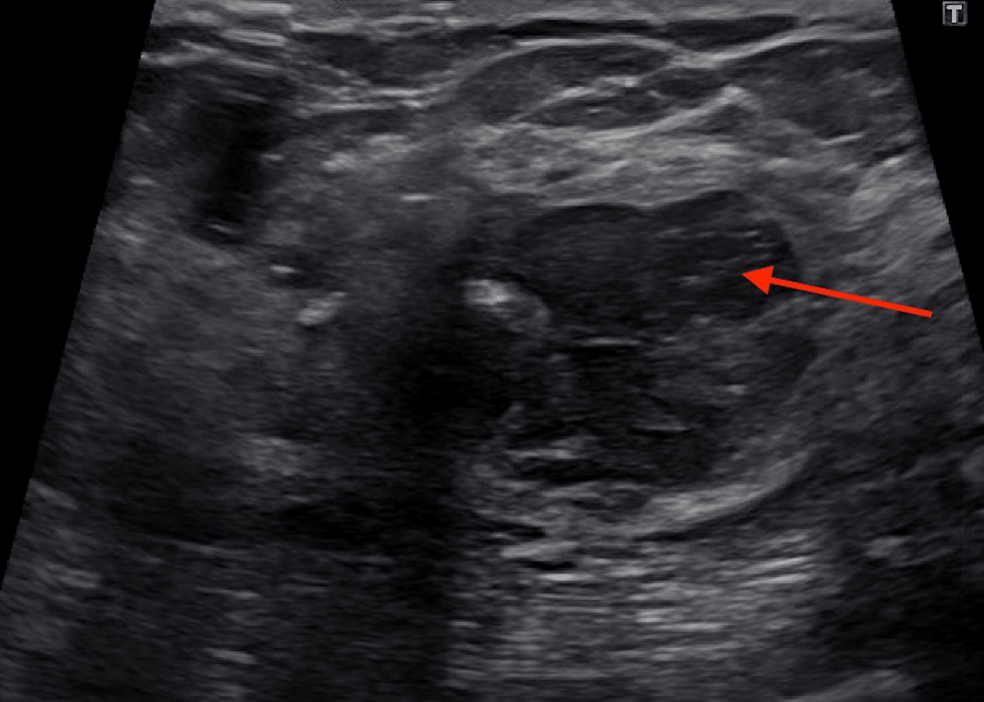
A 2.8 cm hypoechoic mass at the 10:00 position, 5 cm from the nipple, was discovered on a four-year preoperative right breast ultrasound. The mass was subsequently biopsied.
